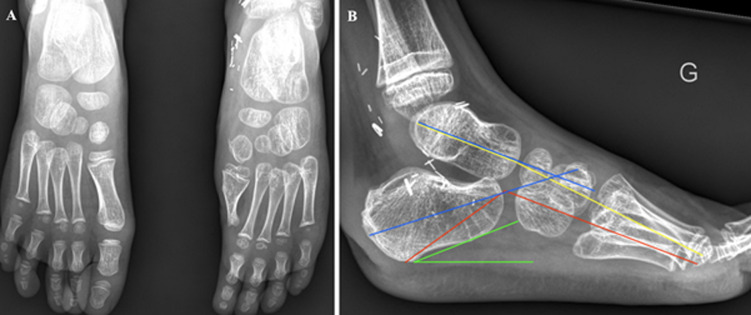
X-ray at 1 year: A) frontal view of both feet; B) weight-bearing lateral view of the left foot with measurement of foot statics parameters (Djian-Annonier angle in red, Méary-Toméno line in yellow, calcaneal slope in green, talocalcaneal divergence angle in blue; all measurements are normal)
